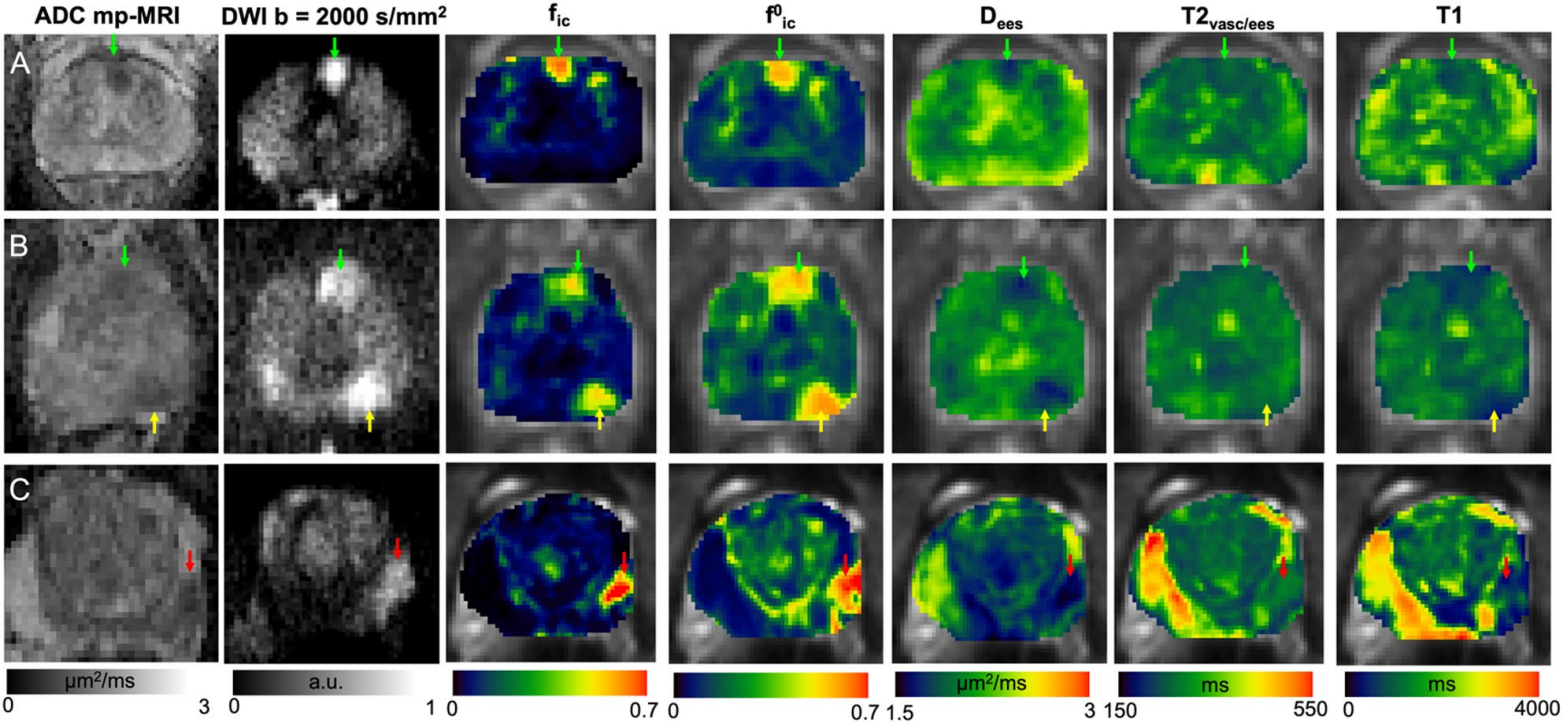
(A–C) Apparent diffusion coefficient (ADC) maps from multi-parametric MRI (mp-MRI); diffusion-weighted image (DWI) at b = 2000s/mm^2^, classic VERDICT intracellular volume fraction f_ic_ and rVERDICT maps (intracellular volume fraction $${\text{f}}^{0}_{{{\text{ic}}}}$$; extracellular-extravascular apparent diffusion coefficient D_ees_; vascular/extracellular-extravascular T2 relaxation time T2_vasc/ees_; T1 relaxation time) for three exemplar patients with different PCa: (A) age late 50’s, PSA 4.78 Gleason 3 + 3 MCCL 7 mm; lesion in the anterior gland; (B): age late 60’s, PSA 5.21 left posterior lesion Gleason 3 + 4 MCCL 14 mm, left anterior lesion Gleason 3 + 3; (C): age late 60’s, PSA 8.68 Gleason 4 + 3, MCCL 10 mm; lesion in the left peripheral zone. Green arrows indicate Gleason grade 3 + 3, yellow arrows Gleason grade 3 + 4 and red arrows Gleason grade 4 + 3.
